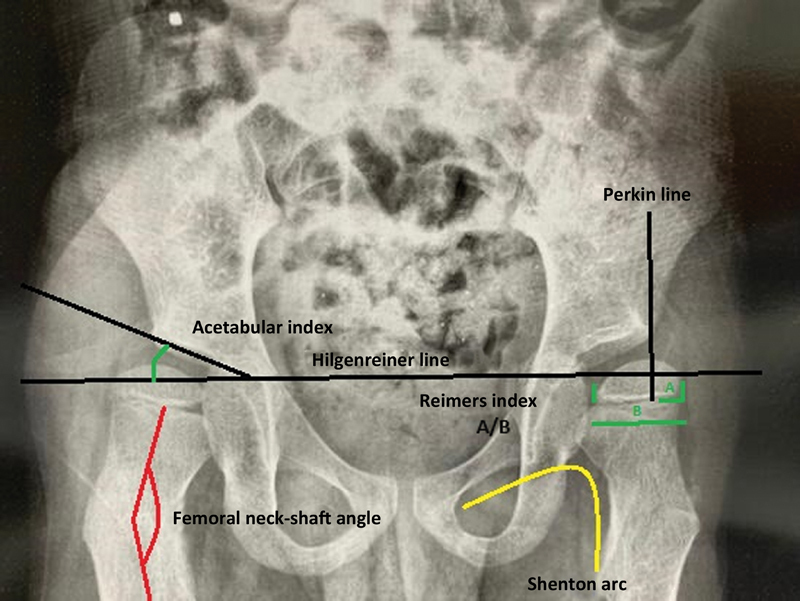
Anteroposterior pelvic radiograph with radiographic measurements.
